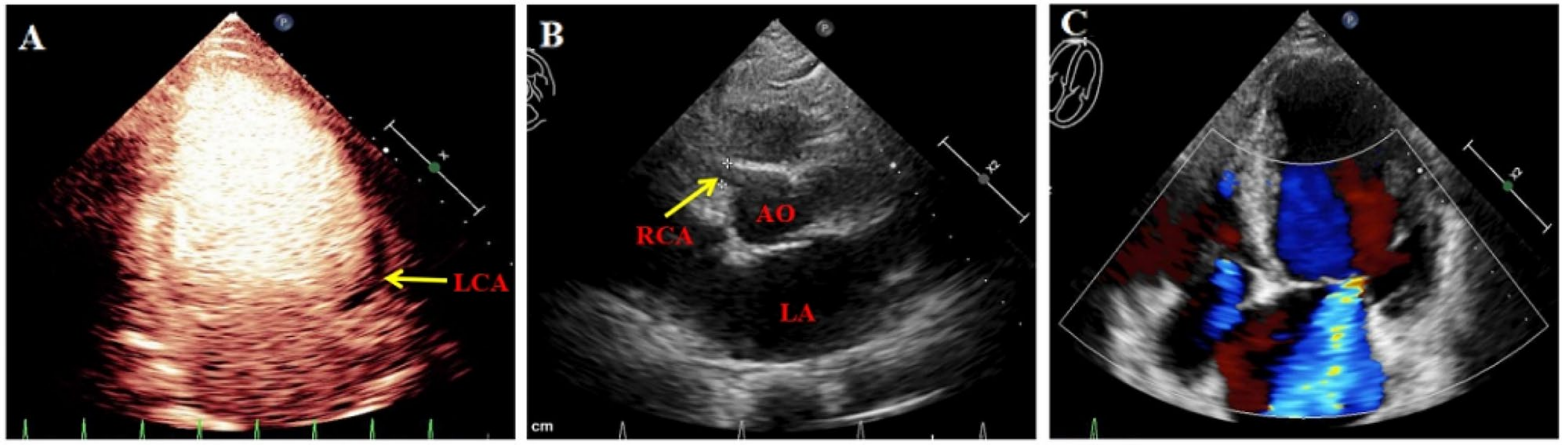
Preoperative color Doppler echocardiography. A The left coronary artery (yellow arrow) visible in the left posterior lateral wall of the middle part of the pulmonary artery. B Dilation of the right coronary artery (yellow arrow) originating from the right aortic sinus; C: Star point blood flow signal can be seen in the myocardium of ventricular septum and lateral wall of left ventricle, PW detection and diastolic phase are the main blood flow signal. AO: aorta, LA: left atrium, LCA: left coronary artery, RCA: right coronary artery
